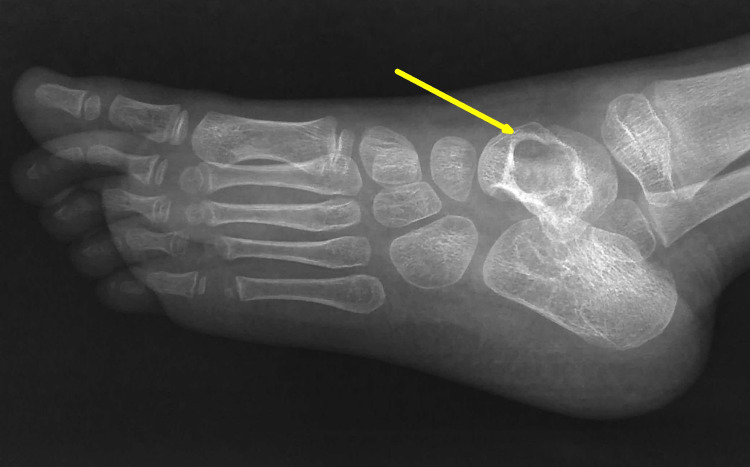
Plain x-ray of left ankle lateral view at presentation The x-ray showed an isolated lytic lesion in the talus bone with a sclerotic rim without any cortical breach (yellow arrow).
